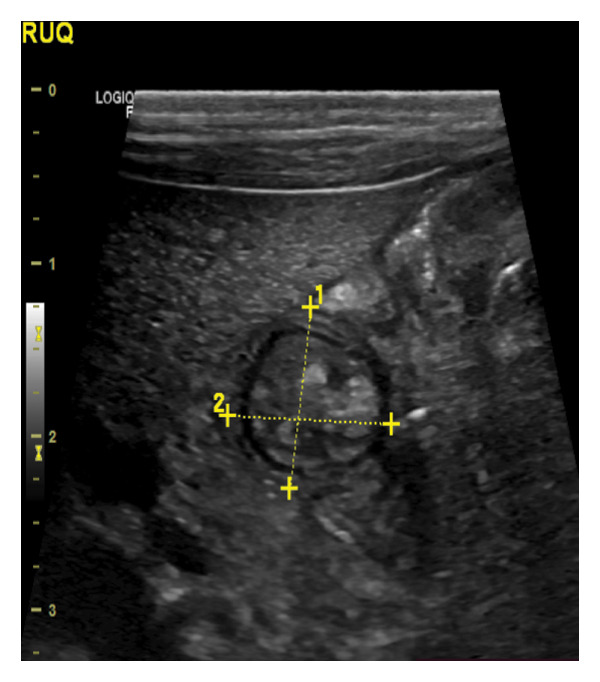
Ultrasound demonstrated focal ileocolic intussusception involving the ileum, cecum, and right colon. The intussuscepted segment measured approximately 2.5 × 1 × 1.1 cm, with the right colon up to the hepatic flexure serving as the intussusceptum. Terminal right‐colon loops showed wall thickening but preserved vascular flow. The intussusception occupied most of the right abdomen, with no free fluid seen between the intussuscipiens and intussusceptum.
